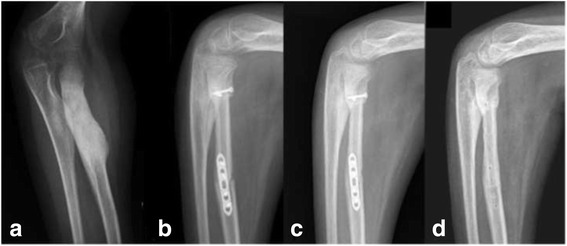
Extended osteoid osteoma of the left proximal radius in a 9-year-old male patient a Postoperative conventional X-rays 3 months after segmental resection b The single strut was fully integrated 5 months after surgery and exhibits hypertrophy at its junctions c Plate removal was performed 7 months after the initial surgery d
